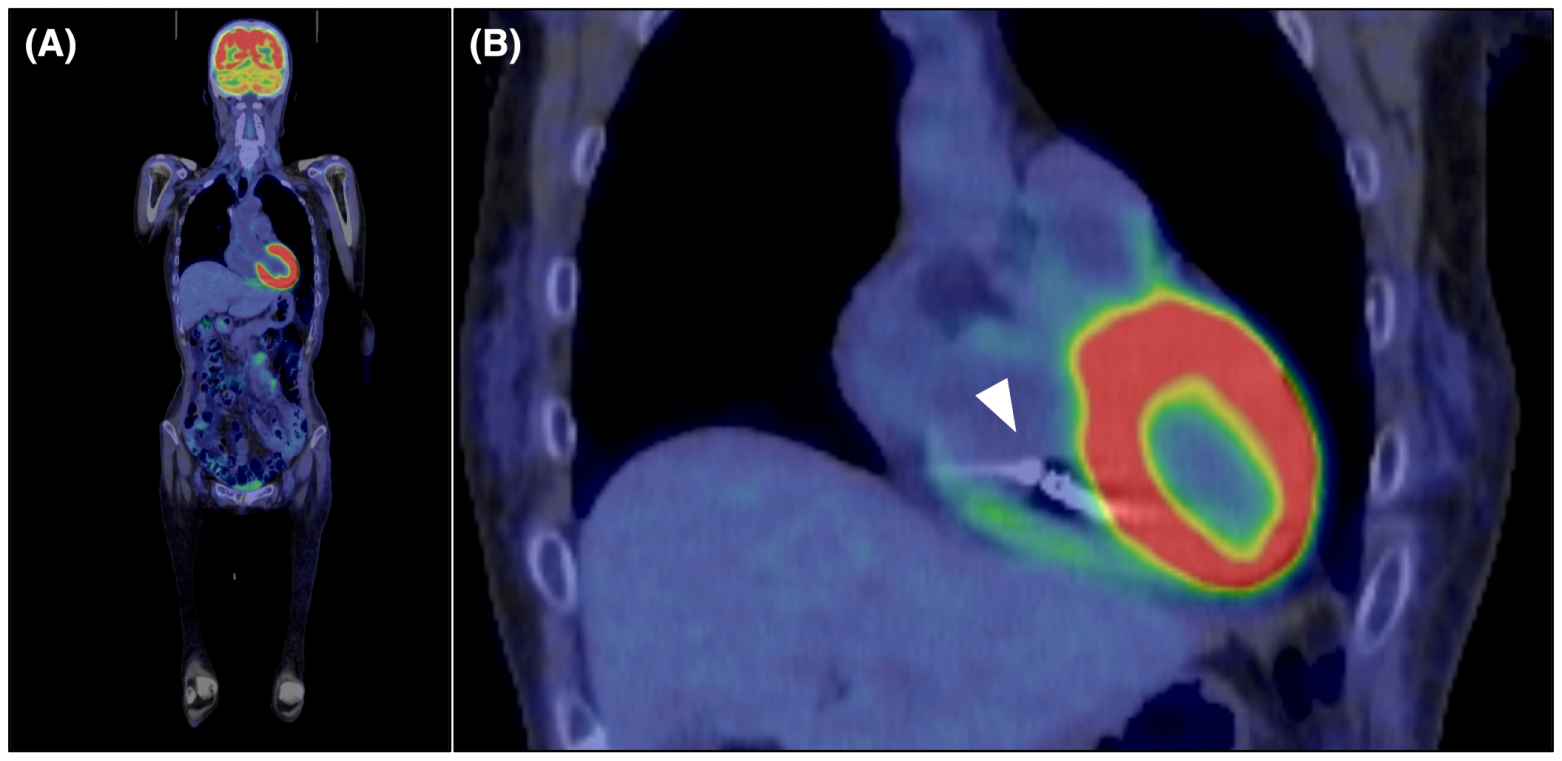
[^18^F]‐FDG‐PET/CT images 7 months after the start of chemotherapy. (A) Overall image shows physiological accumulation in the heart. No abnormal accumulations suspicious of lymphoma exacerbation are observed. (B) A magnified image of the right ventricular apex reveals no abnormal accumulation around the leadless pacemaker body (white arrowhead). [^18^F]‐FDG‐PET/CT, positron emission tomography/computed tomography imaging with [^18^F]‐fluorodeoxyglucose.
